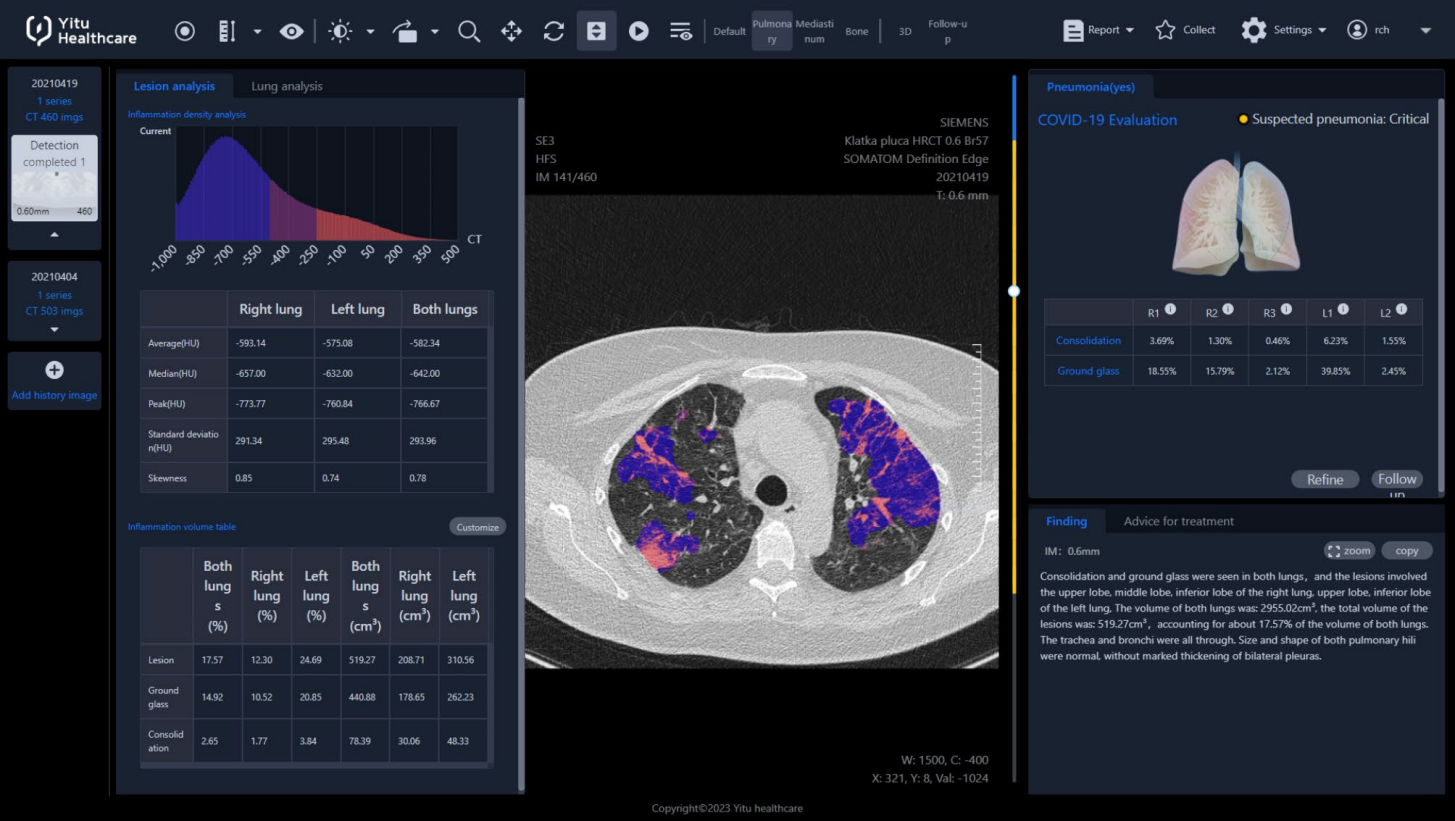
The final report from the automatic analysis of HRCT by AI. Inflammation regions are marked in color depending on attenuation values in Hounsfield units; for example, the areas of ground glass are shown in blue
